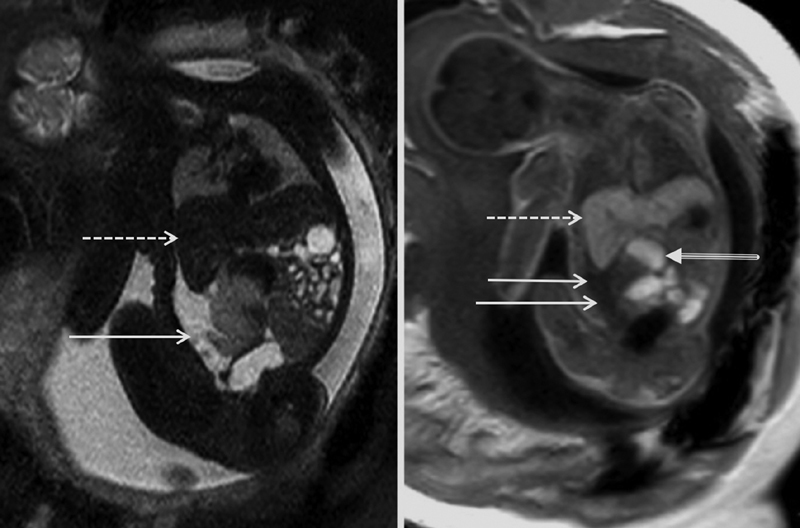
Fetal MRI, coronal slices (T2-sequence on the left side, T1 on the right side): the abdominal mass (white arrows) appears as an intermediate hyperintense T2-lesion and a hypointense to isointense T1-lesion. Visualization of some adjacent dilated bowel loops with meconial content (tri-line arrow). The dotted arrows show the liver. MRI, magnetic resonance imaging.
